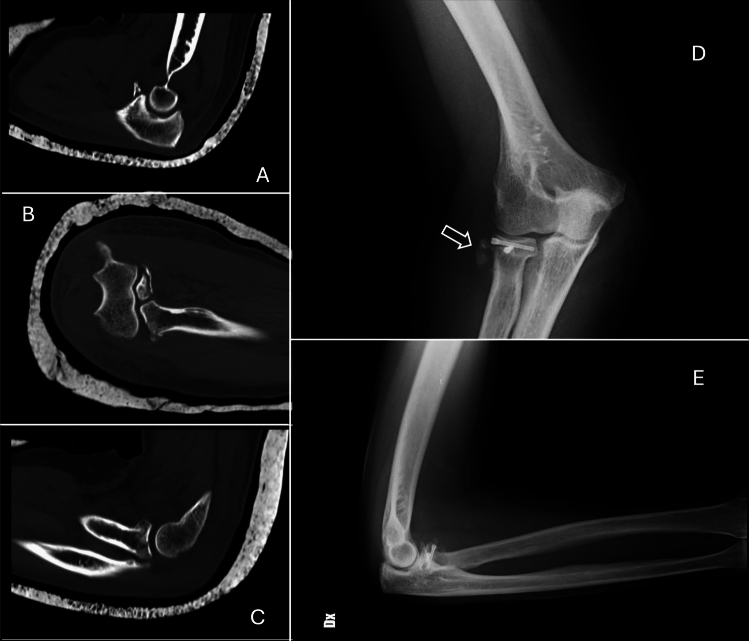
A series of images depicting a patient treated for the terrible triad injury. Images A and B display sagittal and coronal CT scans, respectively, showing a fracture of the coronoid process. Image C presents a sagittal CT scan revealing a fracture of the capitellum. Although the elbow dislocation is part of the terrible triad injury, it is not dislocated in these images but rather stabilized in a cast. Images D and E are postoperative anteroposterior (AP) and lateral radiographs. Note the use of an HCS screw for fixation at the radial capitellum, with heterotopic calcification of the adjacent soft tissues (indicated by the white arrow)
